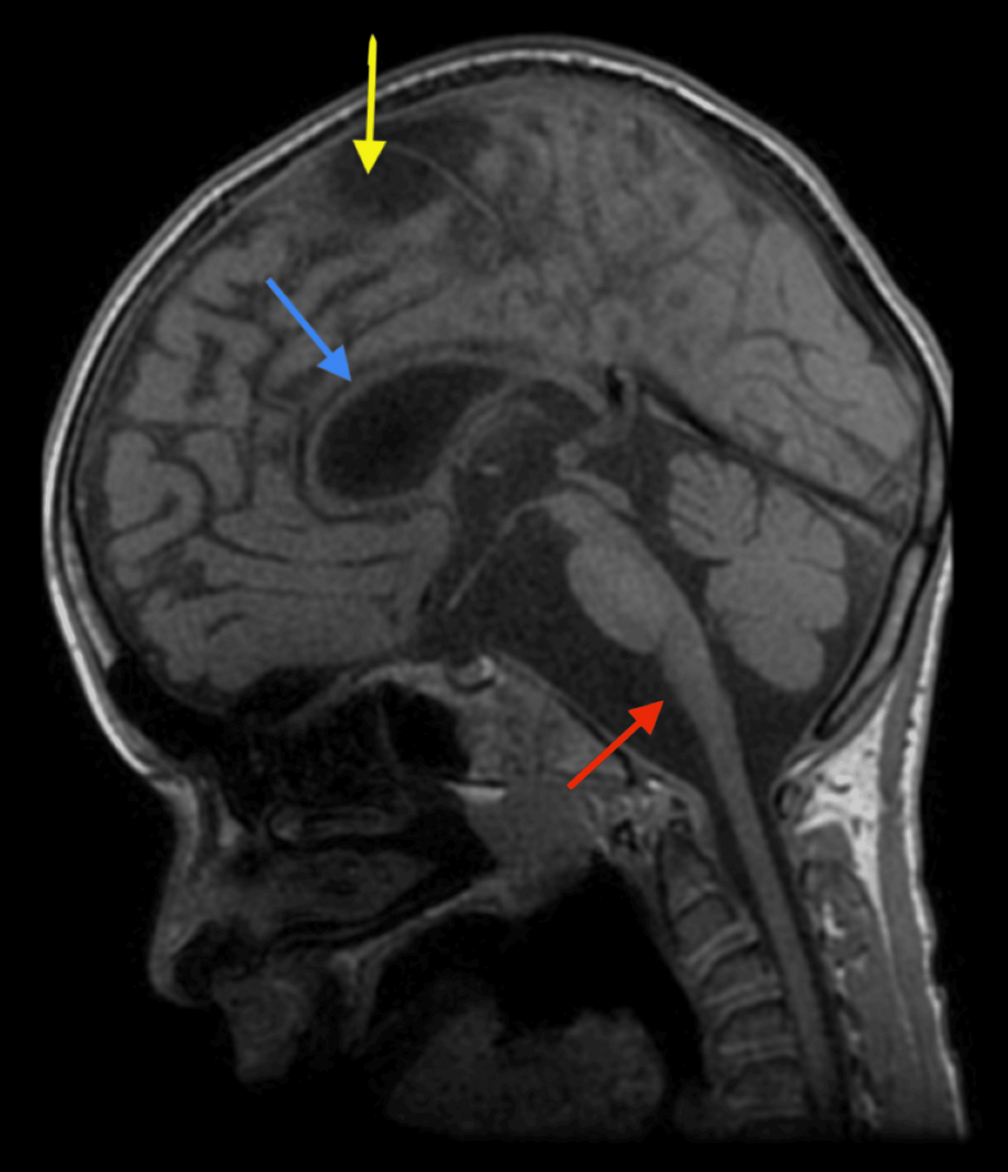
Sagittal T1-weighted imaging The corpus callosum is severely thinned throughout (blue arrow). The brainstem is slender, with large cerebrospinal fluid (CSF) spaces, particularly anterior to the medulla oblongata (red arrow). The foramen magnum is normally sized. An incidental note is made of a right parietal arachnoid cyst at the vertex (yellow arrow).
